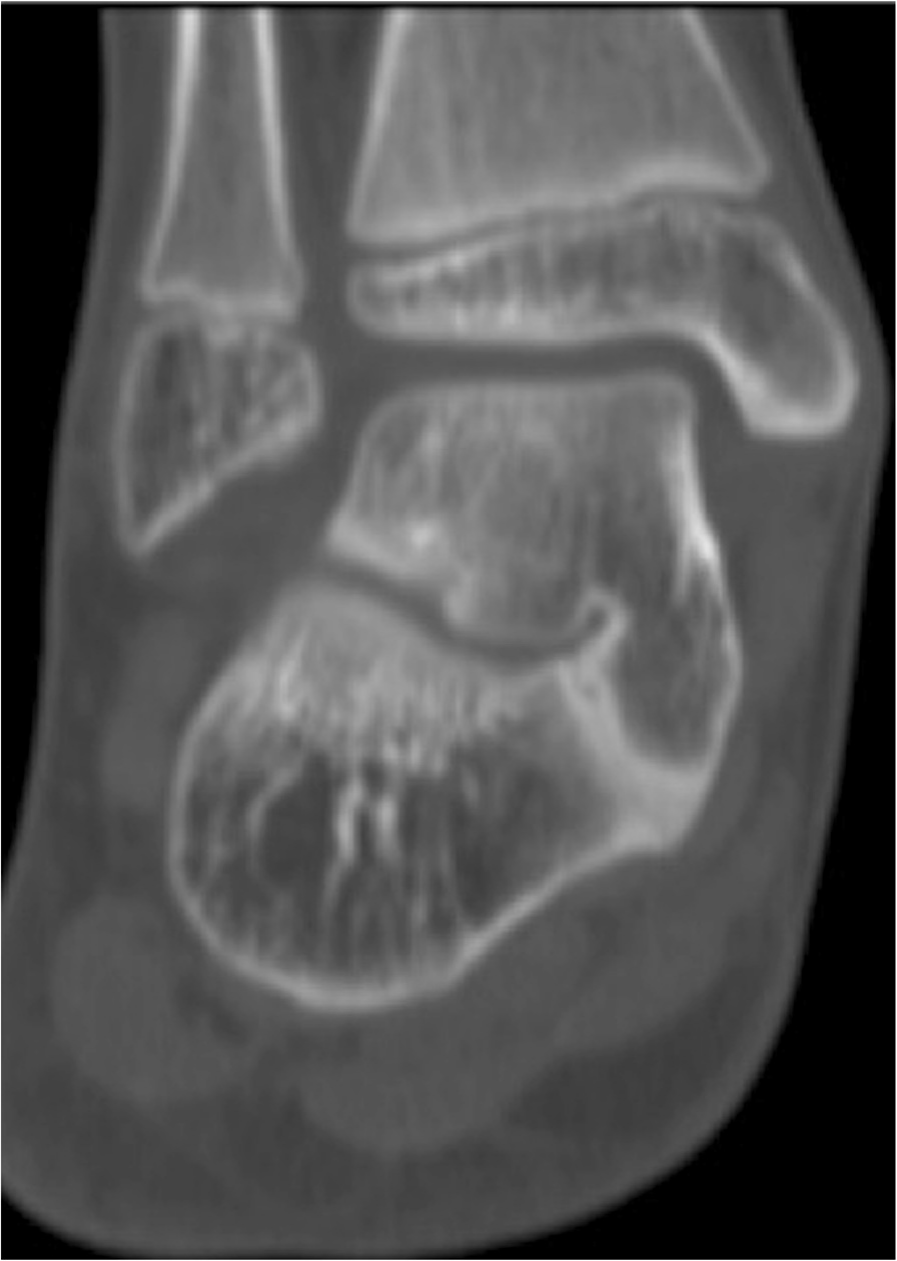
The image of CT shows a bony talocalcaneal coalition.
